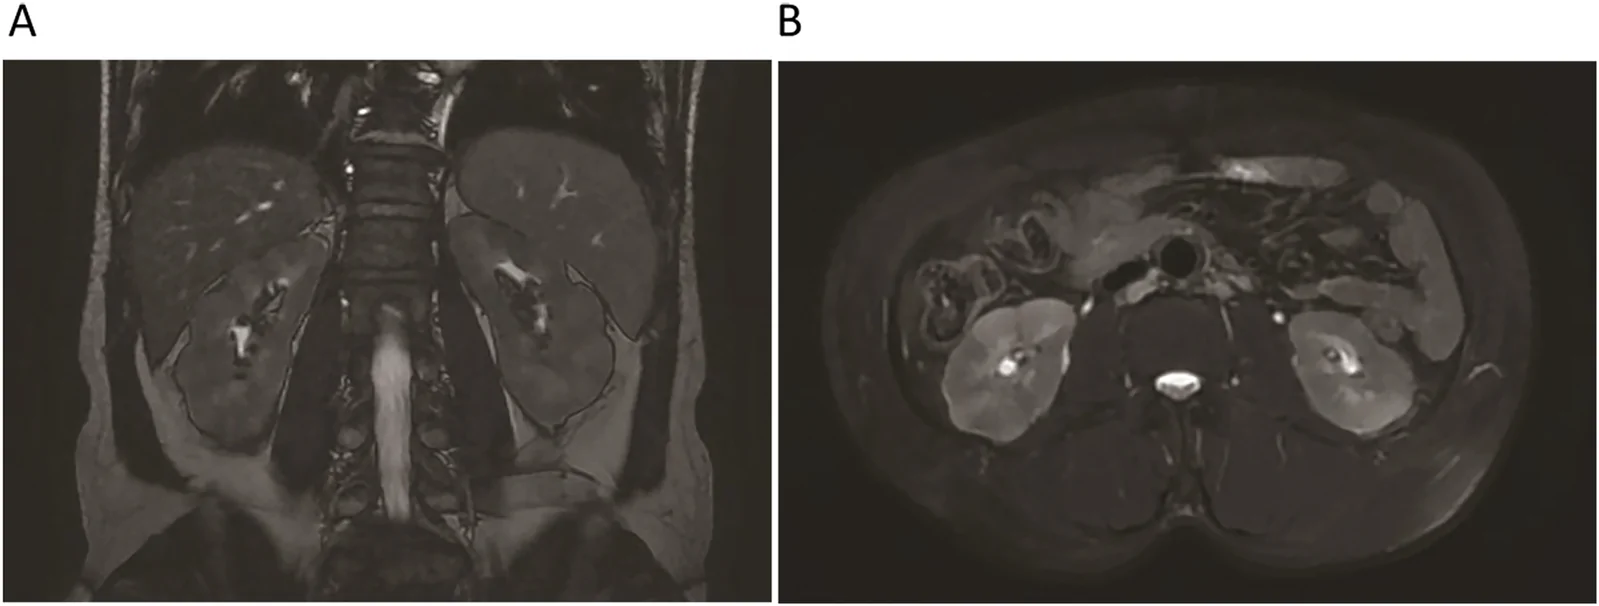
Magnetic resonance imaging of the kidneys; the presence of diffuse signal abnormalities in both kidneys may indicate potential nephritis or nephrotic changes.
